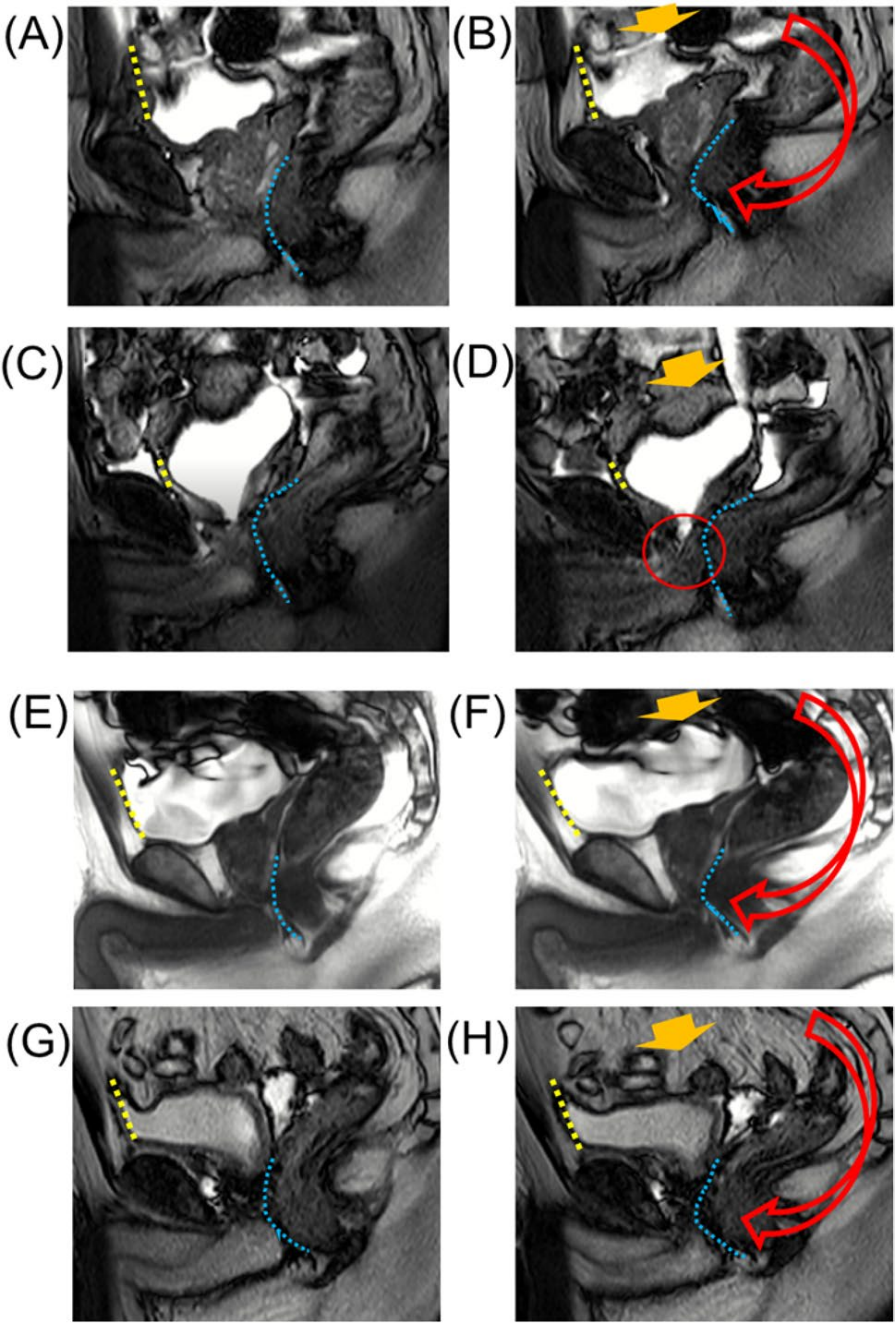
Dynamic mid-sagittal magnetic resonance imaging (MRI) of conventional robot-assisted radical prostatectomy (RARP): preoperatively at rest (A), preoperatively with abdominal pressure (B), postoperatively at rest (C), and postoperatively with abdominal pressure (D). Dynamic mid-sagittal MRI of Retzius-sparing RARP: preoperatively at rest (E), preoperatively with abdominal pressure (F), postoperatively at rest (G), and postoperatively with abdominal pressure (H). Yellow dashed line: attachment between the anterior bladder wall and the abdominal wall. Blue dashed line: the anterior wall of the rectum. (B, F) When applying abdominal pressure (orange arrow), the bladder is compressed caudally. At the same time, the pelvic organs are rotated forward (red arrow) with the anterior wall of the bladder (yellow dashed line) attached to the abdominal wall as a fulcrum, and the membranous urethra is compressed forward (blue dashed line). (C) The attachment of the anterior wall of the bladder moving caudally. (D) When applying abdominal pressure (orange arrow), the bladder is compressed caudally, the bladder neck is enlarged (red circle), and urinary incontinence is observed. (H) When applying abdominal pressure (orange arrow), the bladder is compressed caudally. At the same time, the pelvic organs are rotated forward (red arrow) with the anterior wall of the bladder (yellow dashed line) attached to the abdominal wall as a fulcrum, and the membranous urethra is compressed forward (blue dashed line).
